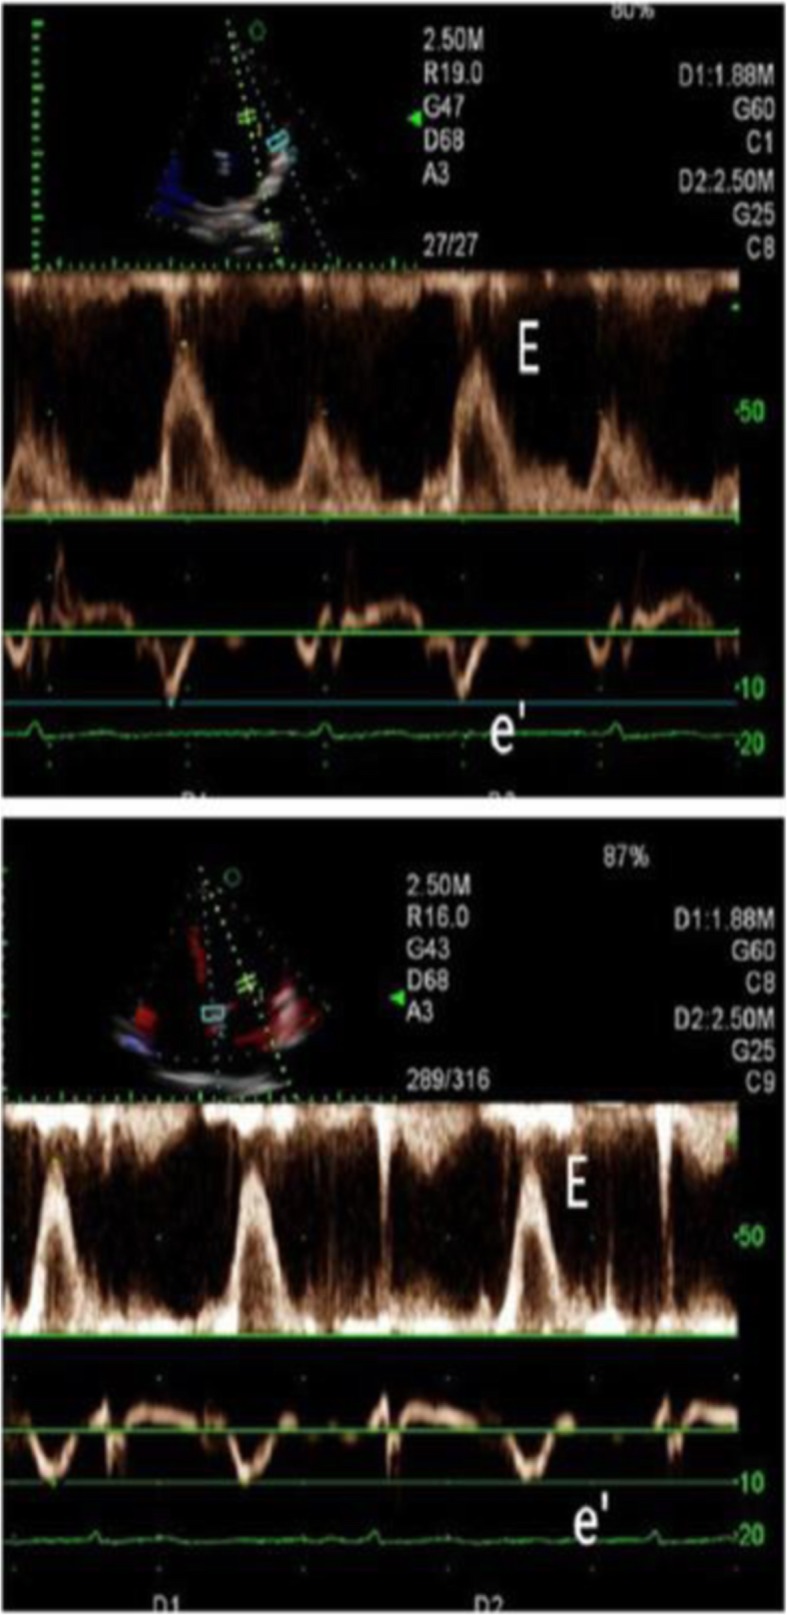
Measurement of transmitral inflow and the mitral annular velocity using a dual Doppler system. A total of 2 pulsed sample volumes were positioned between the tips of the mitral leaflets and the base of the left ventricular lateral wall or interventricular septum, and E and e’ were measured
